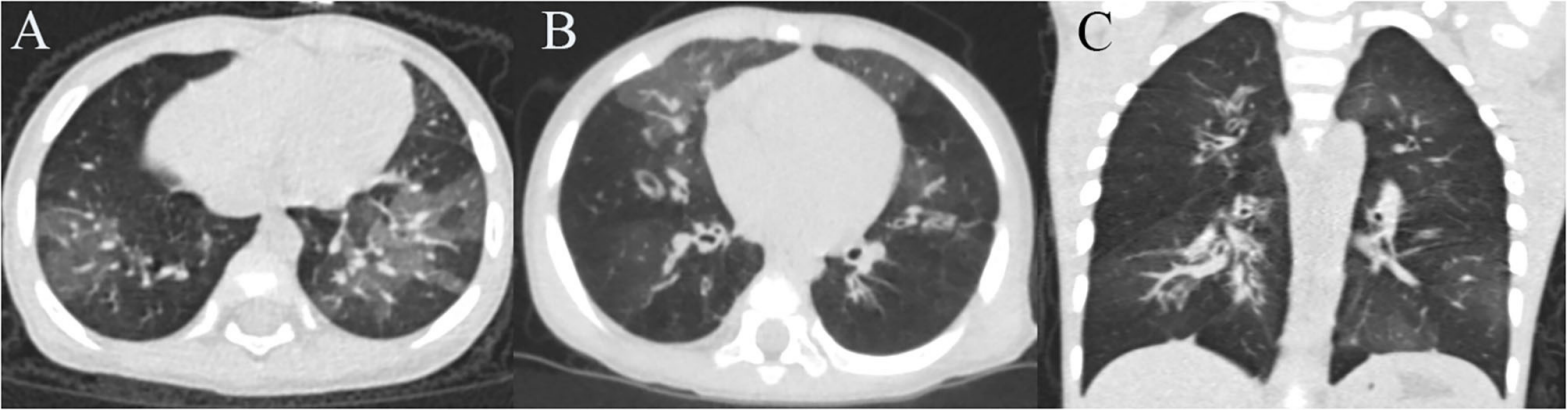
Chest HRCT scans of a 16-month-old girl and a 8-month-old boy with PIBO. (A) Mosaic perfusion pattern and bronchial wall thickening of the girl. (B) Mosaic perfusion pattern, bronchial wall thickening and bronchiectasis of the boy (C) 3D reconstruction (the boy).
